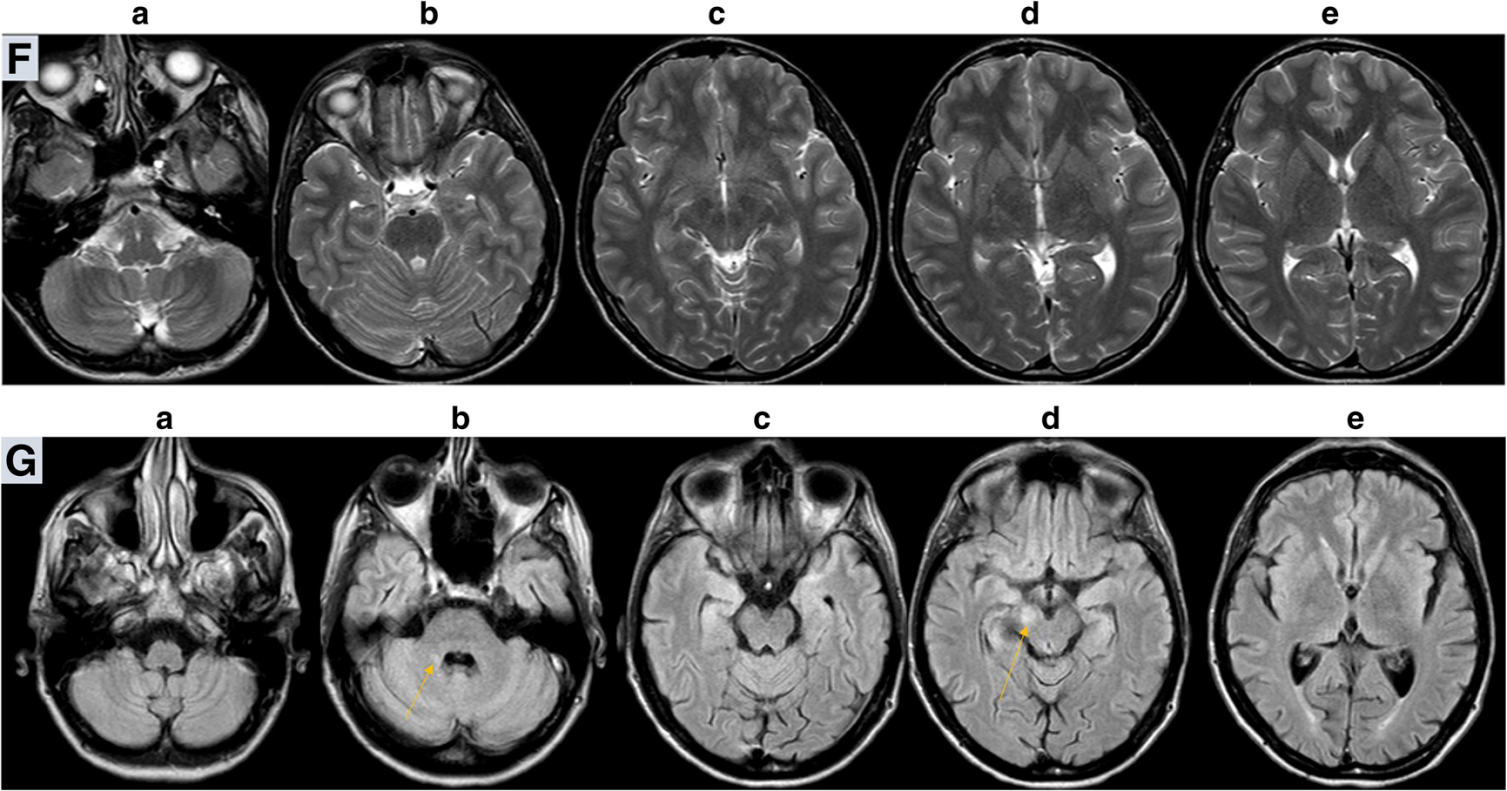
Serial axial T2-weighted brain MR images of patients 1, 2, 3 and family members (FLAIR sequence) bearing m.9185T>C variant of *MTATP6*, and autopsy findings in patient 3. Patient 1. Line A, at LS presentation at the age of 33: bilateral, symmetric hyperintensities in medulla oblongata, peripheral parts of pons and midbrain. Line B, on follow-up, 11 months later: a total regression of changes in peripheral parts of pons, presence of the previously detected lesions located in midbrain and in medulla oblongata. Greater degree of lesion demarcation. New symmetric lesions (T2-weighted hyperintensities) bilaterally within middle cerebellar peduncles. Patient 2. Line C, at LS presentation at the age of 5: high signal of the medulla oblongata (a), midbrain (b, c) and periaqueductal area (d). Small cystic-like lesions in the heads of the caudate nuclei (e). Line D, on the follow-up, at the age of 8: a partial but significant reduction in the severity of changes. Patient 3. Line E, at LS presentation at the age of 9: poor demarcation hyperintensities located in medulla oblongata (a), and midbrain (b, c, d); symmetric high signal lesions of the lower parts of striatum. Healthy brother of patient 3. Line F, normal MRI (a–e). Mother of patient 3. Line G, hyperintensive lesions in the posterior part of right middle cerebellar peduncle (b) and right cerebral peduncle (d), without changes in the medulla oblongata, periaqueductal region (a, c) and basal nuclei (e)
